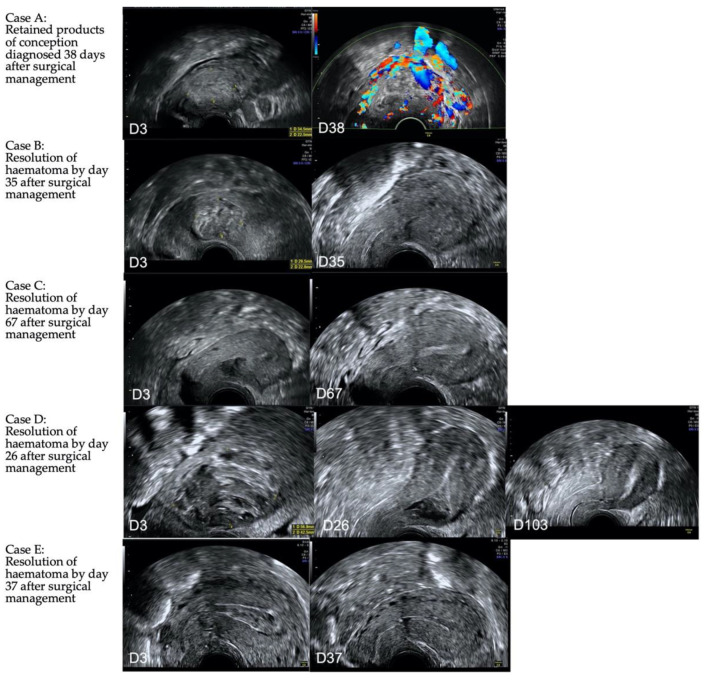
Longitudinal ultrasound images of five cases (case A–E) managed surgically with cerclage and balloon. The initial image was obtained on day 3 (D3) at the time of cerclage removal, and subsequent images are obtained between day 26 and 103 post surgical management. In 4/5 the haematoma present on day 3 resolves, whereas in one (A), retained products of conception is diagnosed at day 38 post surgery.
